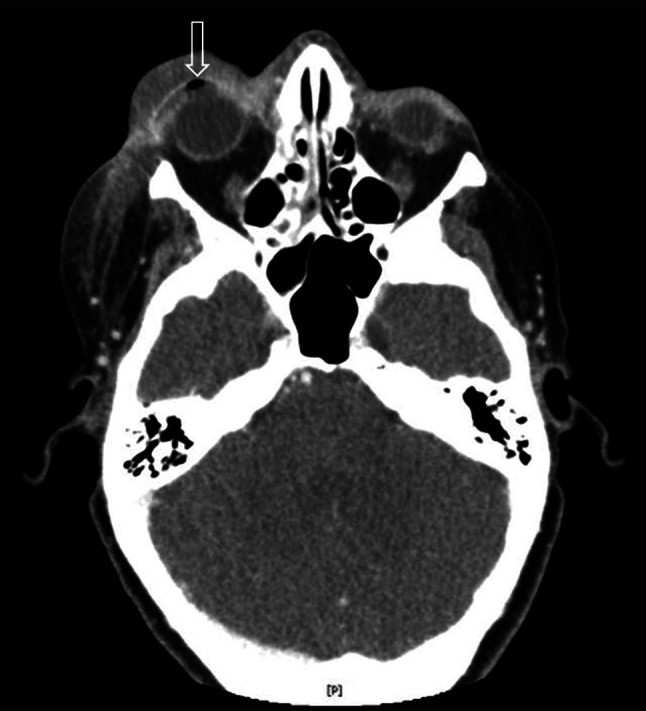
Non-contrast computed tomography at the initial presentation. Bilateral ethmoid sinus mucosal thickening and diffuse preseptal soft tissue swelling are observed, with enlarged right cervical lymph nodes extending from the periorbital to the anterior temporal region. No intraorbital abscess, postseptal extension, or intraocular abnormality is identified. An apparent gaseous shadow over the cornea (arrow) initially raised concerns regarding a gas-forming infection but was subsequently interpreted as trapped air related to severe eyelid edema rather than true soft-tissue gas
